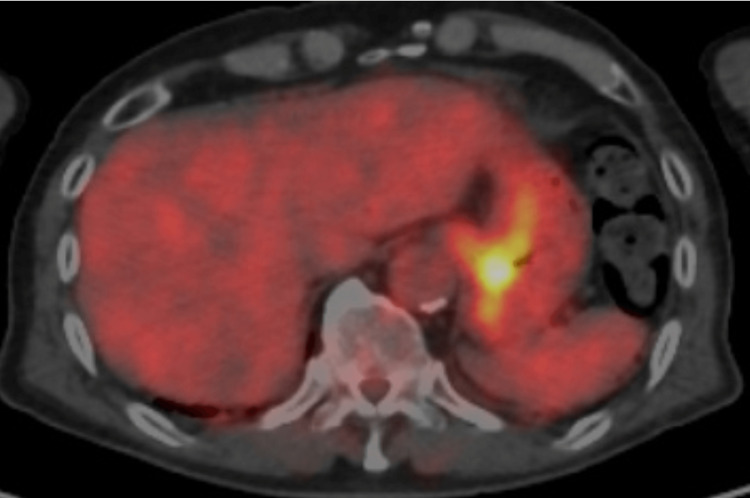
PET/CT findings PET/CT showed avid fluorodeoxyglucose uptake in the cardia 20 months after endoscopic submucosal dissection. PET/CT, positron emission tomography/computed tomography
